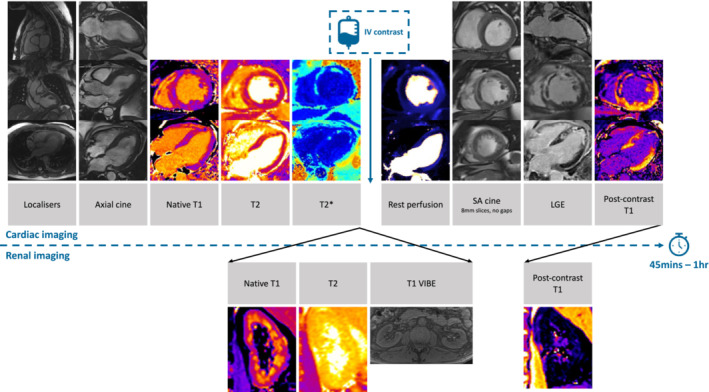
Cardiovascular magnetic resonance imaging protocol outline. Cardiovascular magnetic resonance imaging will be performed pre‐randomization and at 24 weeks on a single 1.5 Tesla Siemens MAGNETOM Avanto scanner. IV, intravenous; LGE, late gadolinium enhancement; SA, short‐axis; VIBE, volumetric interpolated breath‐hold examination.
